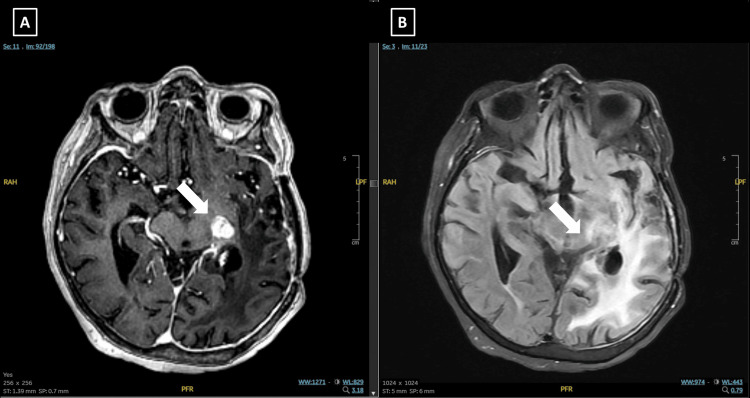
Diagnostic MRI images of Case 3. (A) Axial T1-weighted post-contrast (T1C+) MRI demonstrating a recurrence involving the left frontal and parietal lobes. (B) Axial T2 fluid-attenuated inversion recovery (FLAIR) image corresponding to the lesion.
